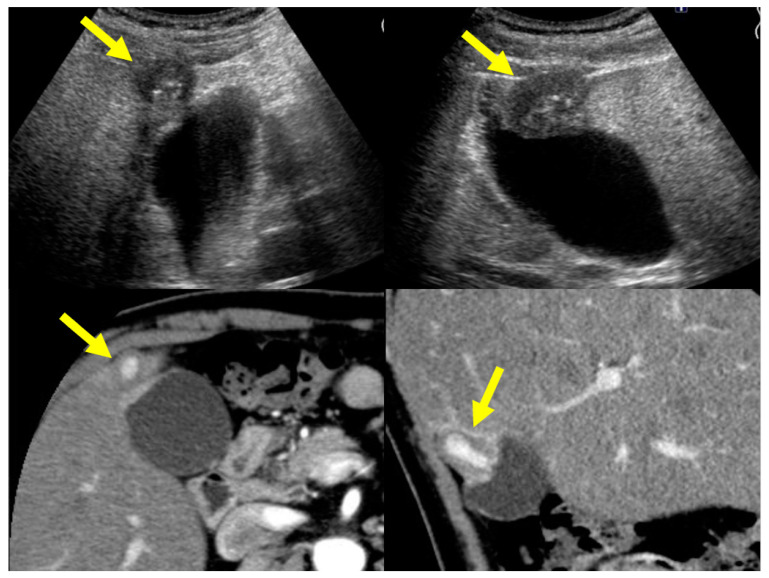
US (protruding type A) and CT (protruding type A) findings of a case with a localized type of IgG4-sclerosing cholecystitis (arrows).
